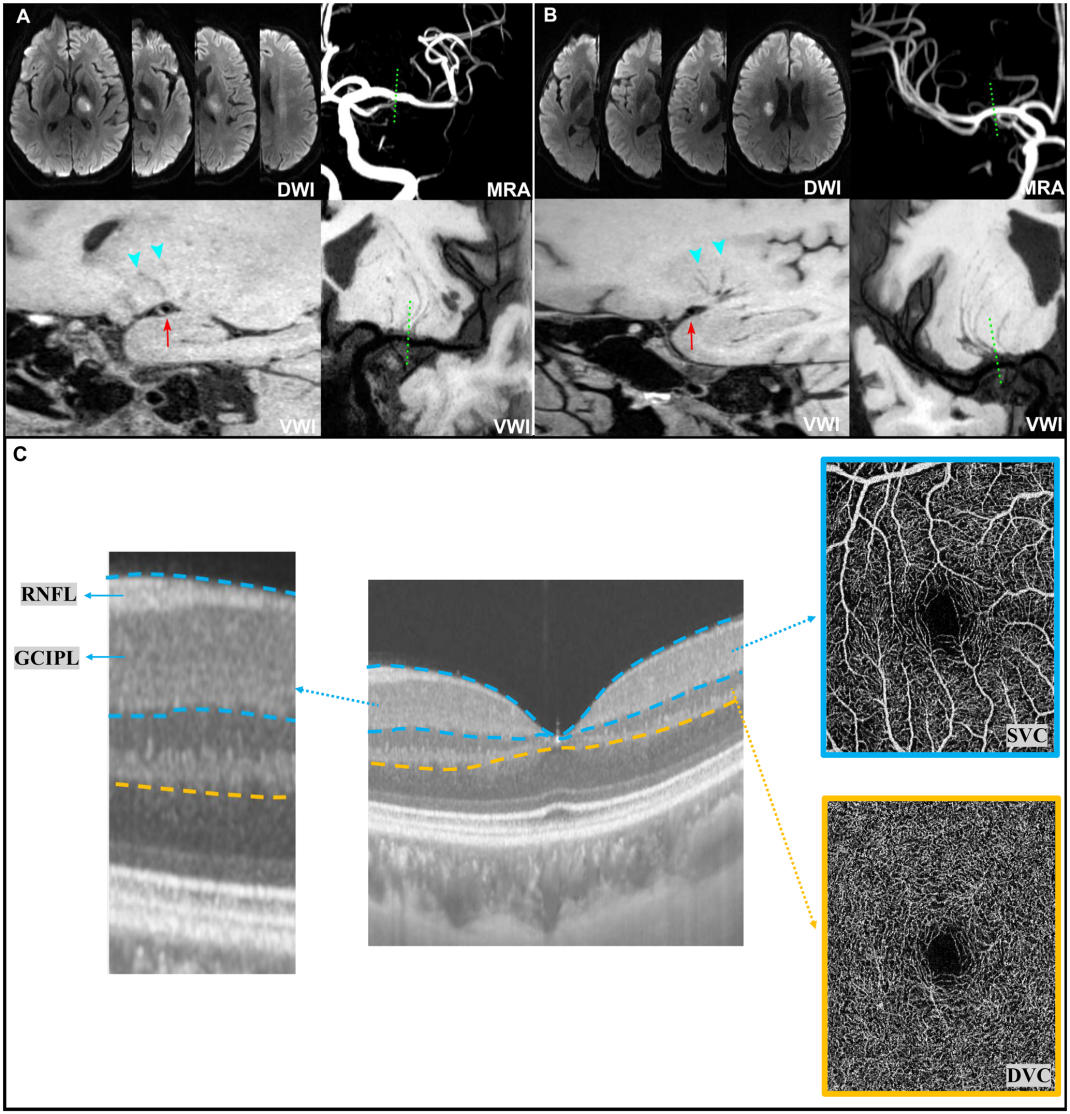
Classification of the etiological subtypes of RSSI based on high‐resolution magnetic resonance vessel wall imaging (VWI), and retinal microvascular and structural segmentation. (A) Branch atheromatous disease (BAD) DWI showed proximal RSSI involvement in 3 consecutive axial slices. Magnetic resonance angiography (MRA) showed no stenosis on the relevant middle cerebral artery (MCA). Curved multi‐planar reconstruction (curved‐MPR) showed a culprit plaque (red arrow) adjacent to the corresponding lenticulostriate arteries (LSAs) origins (dashed lines), demonstrated by the magnified image on cross‐section view with pruning LSAs (arrowheads). (B) Intrinsic cerebral small‐vessel disease‐related lacunar infarction (CSVD‐related LI) DWI showed distal RSSI involvement in 2 consecutive axial slices. Curved MPR showed no culprit plaque (red arrow) on the relevant MCA (dashed lines), demonstrated by the magnified image on cross‐section view with traceable LSAs (arrowheads). (C) Segmentation and representative images of retinal angiograms (retinal vascular plexuses) and retinal structural thicknesses. DVC, deep vascular complex; GCIPL, ganglion cell‐inner plexiform layer; RNFL, retinal nerve fiber layer; SVC, superficial vascular complex.
